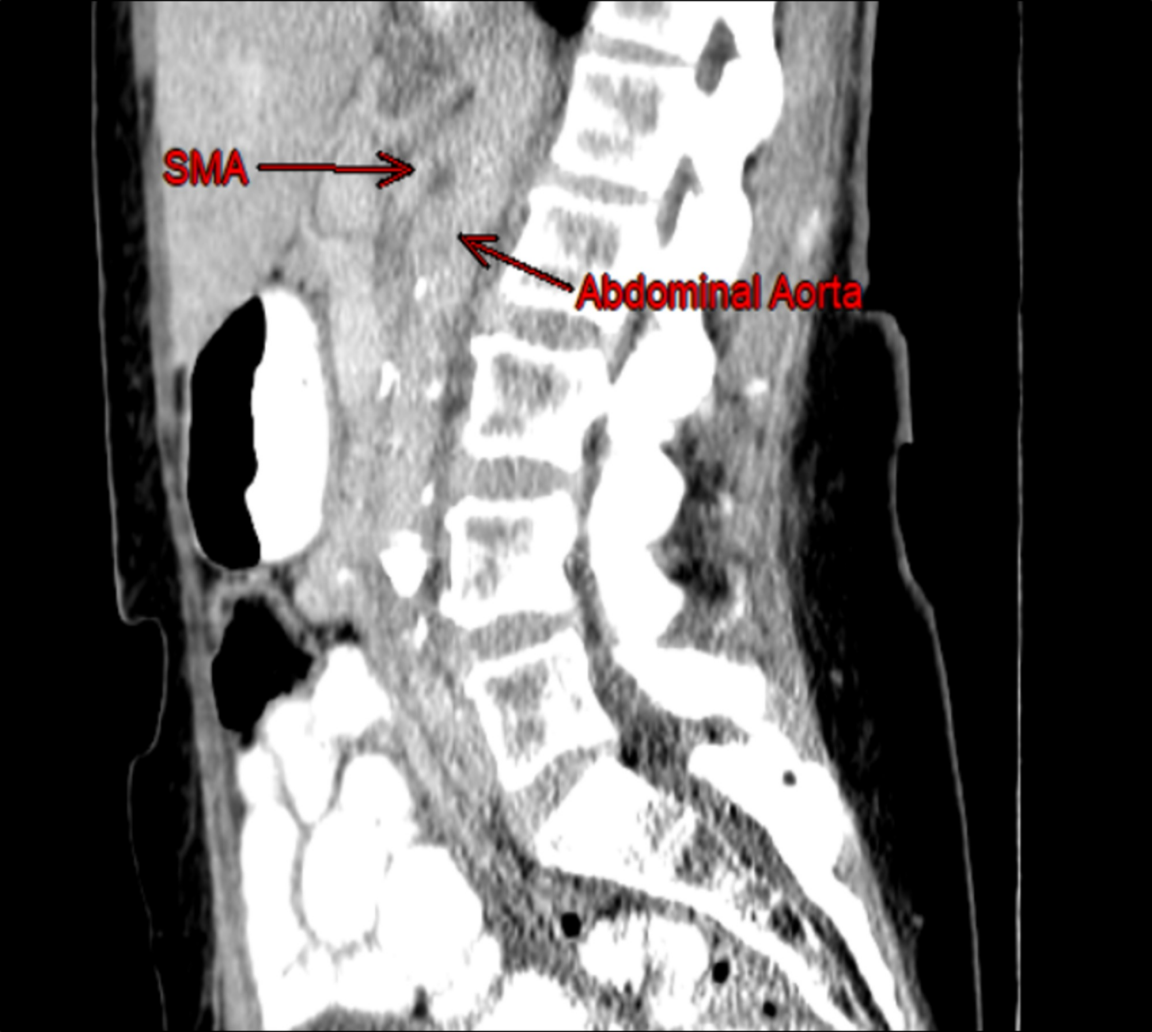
SMA and the abdominal aorta. Sagittal CT scan image is identical to Figure 1. The angle marking has been removed for unobstructed visualization of the vessels. SMA: superior mesenteric artery; CT: computed tomography.
